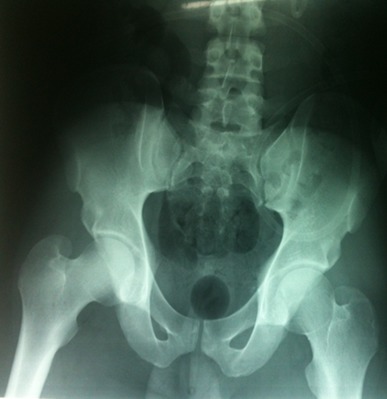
Post reduction radiograph showing the right hip congruency
